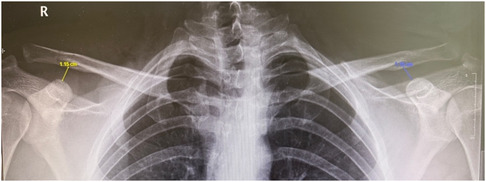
Postoperative anteroposterior radiograph of the right shoulder following acromioclavicular and coracoclavicular ligament reconstruction using the all‐suture technique. The final construct shows reduction and alignment of the acromioclavicular joint without metallic hardware.
